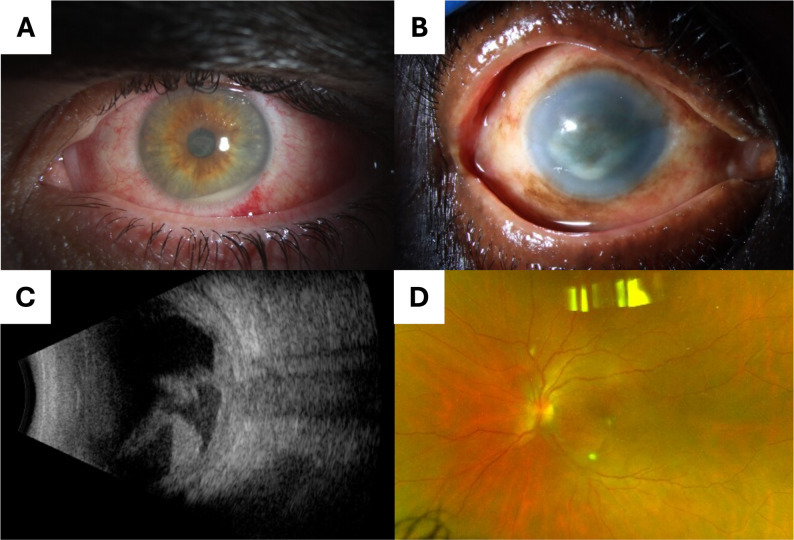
Clinical findings of patients presenting with endogenous endophthlamitis. (A) A 40-year-old male who presented with conjunctival injection and hypopyon from urinary catheter-associated endogenous endophthalmitis that grew *Staphylococcus aureus*. (B) A 79-year-old female who presented with corneal infiltrate and anterior cell fibrin that was found to have soft-tissue abscess-associated endogenous endophthalmitis that grew *Klebsiella pneumoniae*. (C) Ultrasonic findings of dense vitritis, thick membranes, choroidal and retinal thickening and shallow choroidal detachment of patient B. (D) A 31-year-old female with history of intravenous drug use presented with blurred vision. Optos ultra-widefield pseudocolor images of the fundus were performed demonstrating retinal infiltrates OS > OD. Cultures were positive for *Candida albicans*
